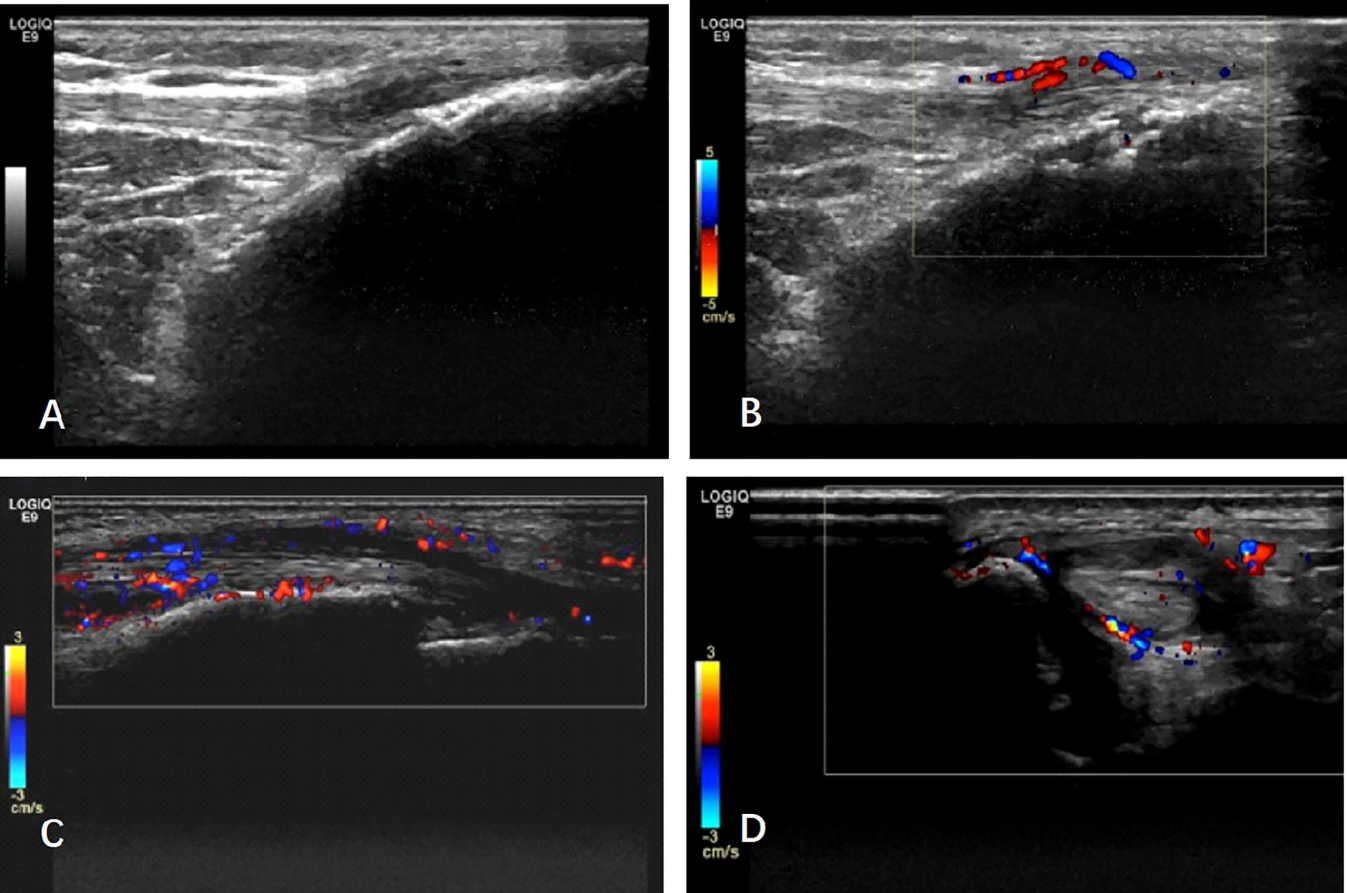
The typical ultrasound image of psoriatic arthritis: (A, B) Enthesitis: (A) longitudinal scan of patellar tendon at its distal insertion into the anterior tibial tuberosity. Note the presence of hypoechoic areas, entheseal thickening together with an enthesophyte. (B) B-mode signs of enthesitis are detectable. The image shows evidence of power Doppler signal at the entheseal area, together with erosion. (C, D) Tenosynovitis: longitudinal and transverse scan of anterior tibialis tendon. Note abnormal anechoic and hypoechoic tendon sheath widening with intense power Doppler signals.
